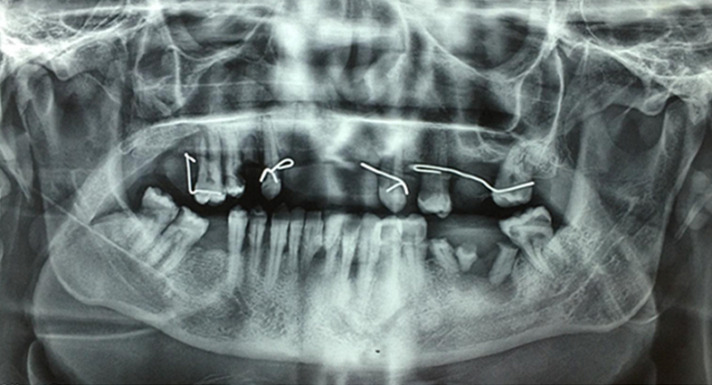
panoramic X-ray showing generalized aggressive perodontitis
